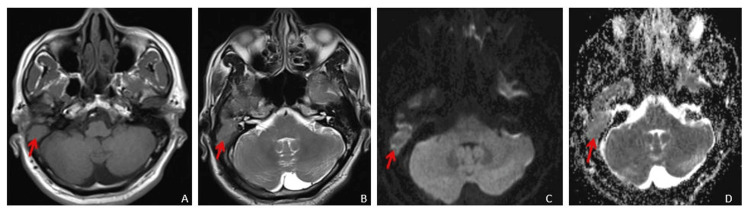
MRI brain axial images (A - T1WI, B - T2WI, C - DWI, D - ADC) showing soft tissue intensity lesion in right middle ear cavity and mastoid air cells causing destruction of the outer mastic cortex, showing minimal diffusion restriction on DWI with low ADC values. MRI: Magnetic resonance imaging; DWI: diffusion-weighted imaging; ADC: apparent diffusion coefficient; T1WI: T1-weighted image; T2WI; T2-weighted image
